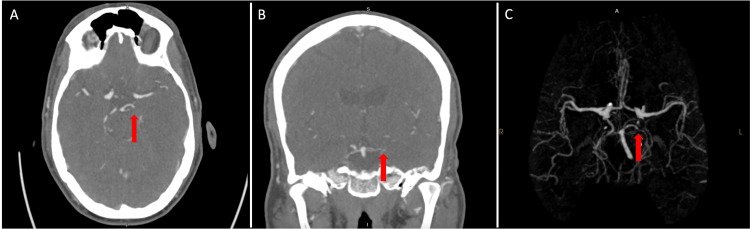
Case 2: Abrupt cutoff of blood flow in the proximal left PCA artery on CT arteriography (red arrow) (A) CT axial view. (B) CT coronal view. (C) Reconstruction of the circle of Willis. PCA, posterior cerebral artery
